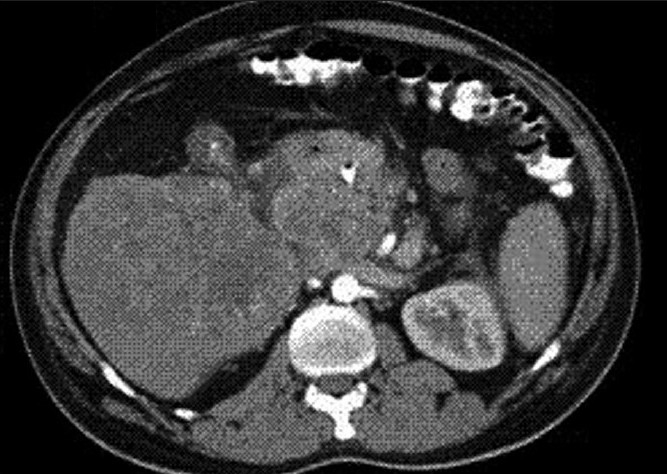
CT abdomen (Transverse section) showing a large caudate lobe liver mass involving the gall bladder and common bile duct at porta with IHBR dilatation
